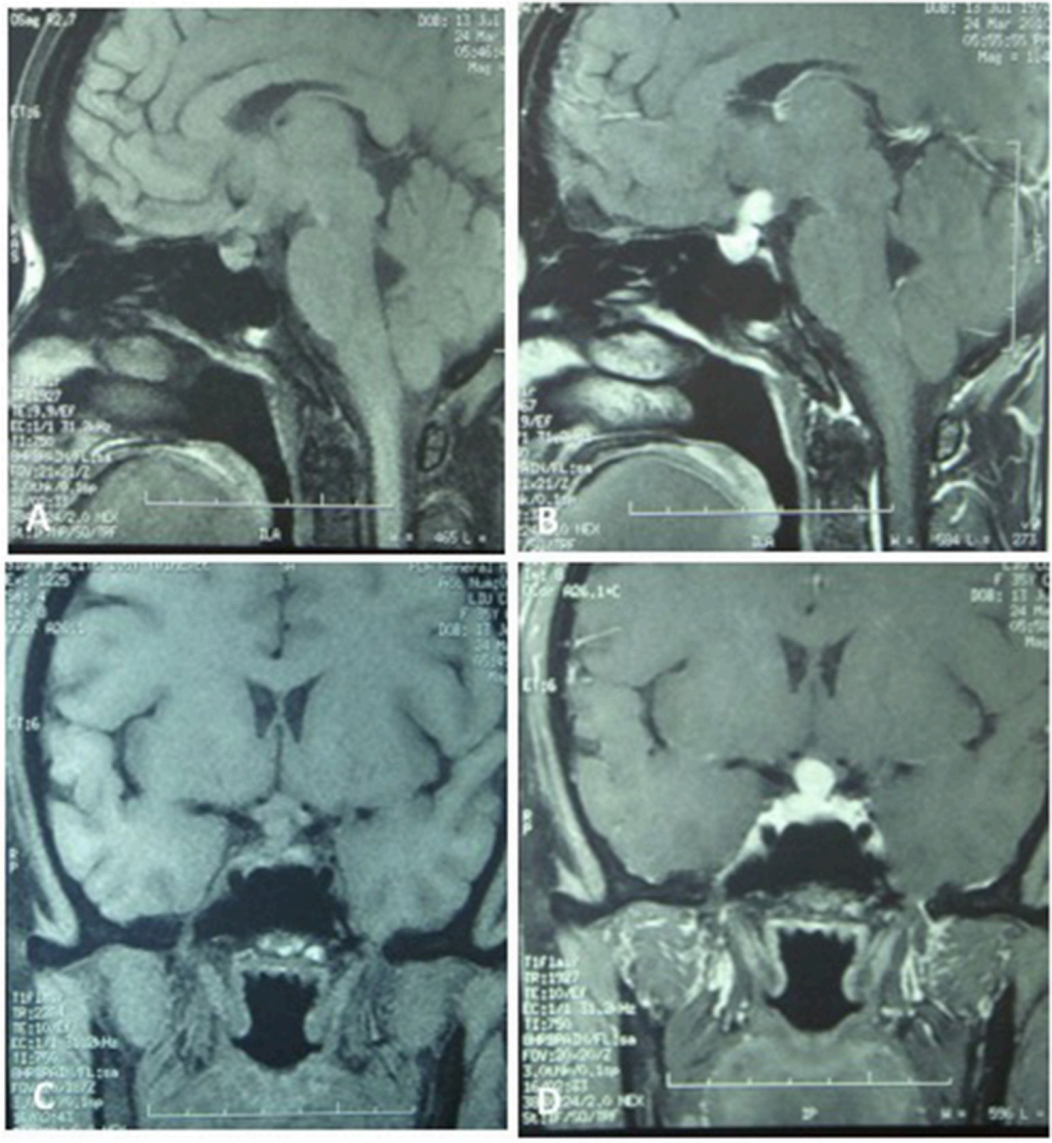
The MRI of the brain and the pituitary. (A,C) No significant expansion of the sella, irregular cystic-solid mixed mass in the hypothalamus region, solid part presented iso-signal, the boundaries between the lesion and pituitary stalk, pituitary are not clear, the optic chiasm was compressed. (B,D) The solid part of the lesion was significantly enhanced, no enhancement in cystic part, no abnormal signal in the brain parenchyma and paranasal sinus.
